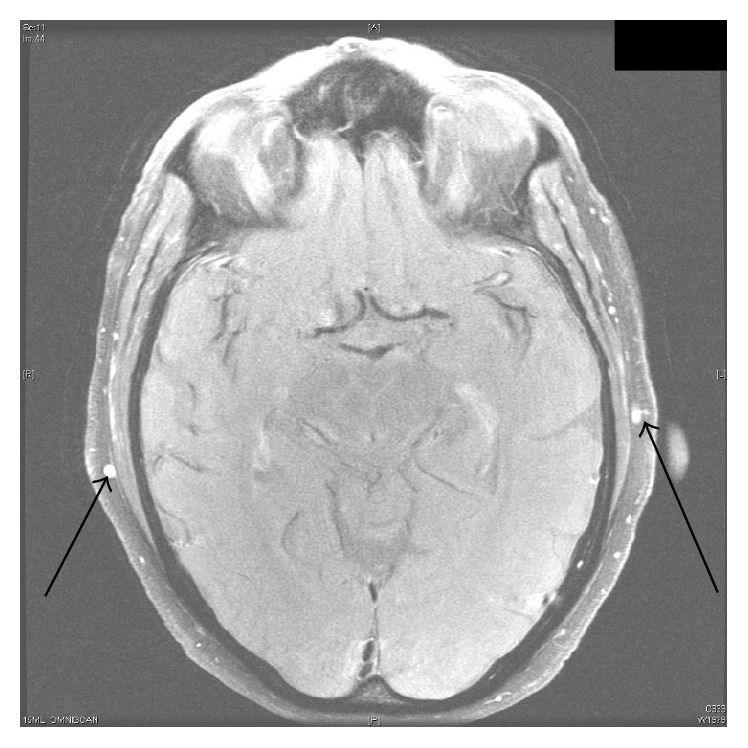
MRI showing mural thickening and enhancement of temporal arteries bilaterally (arrows).
